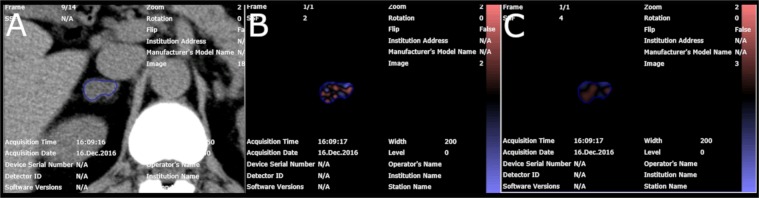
Screen shot of the CT texture analysis software. A polygonal ROI was drawn on the tumor (A). Processed images using Laplacian of Gaussian filters with SSF of 2 mm (B) and 4 mm (C) were automatically generated. The images were displayed using a red or blue scale showing negative or positive pixels, respectively.
